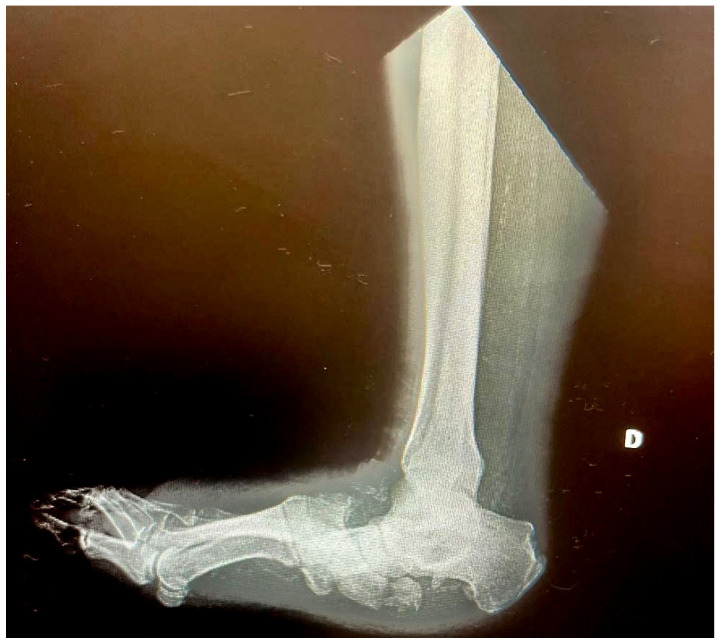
X-ray: Rocker-bottom deformity, dislocation of the tarsometatarsal joints from the second to the fifth rays, along with bony fragmentation and collapse of the midfoot.
